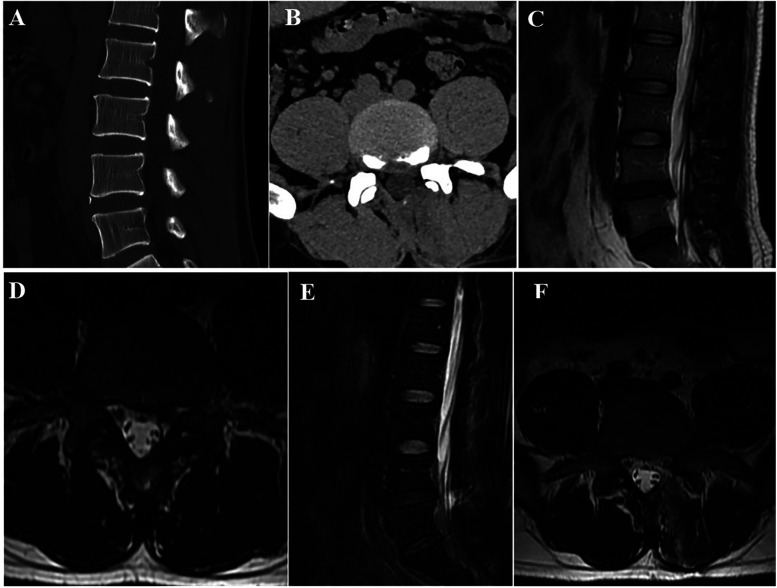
Radiographic data of a patient treated with OSE. (A,B) Transverse and sagittal lumbar CT revealed LDH with severe lumbar lateral recess stenosis at L4/5. (C,D). Lumbar MRI showed the same results as the CT. (E,F) Lumbar MRI after OSE showed that the lumbar lateral recess stenosis was completely opened.
